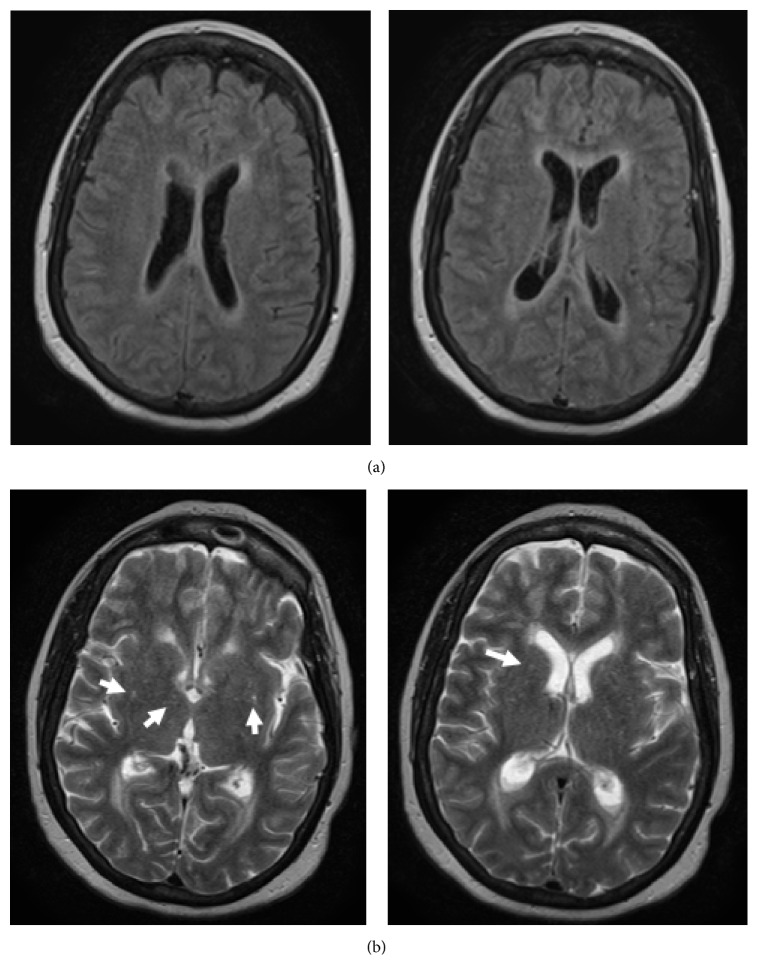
MRI brain taken on hospital day 3. (a) FLAIR enhancement of the ventricles and fluid levels within the posterior lateral ventricles, consistent with ventriculitis. (b) T2 enhanced images showing acute infarcts in the periventricular areas (arrows).
